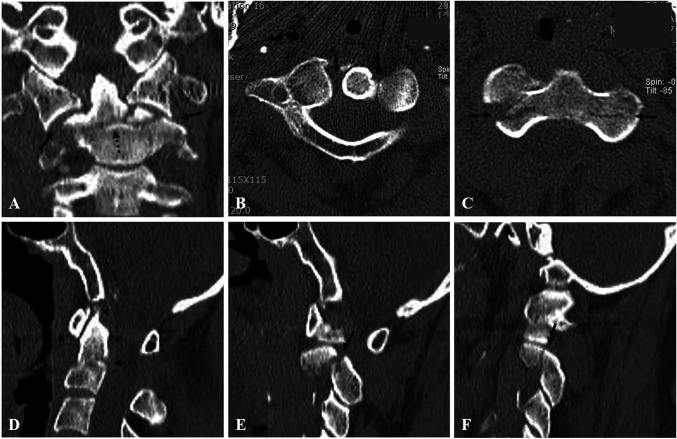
Coronal (A) CT (CT) scan showing a type 3 dens fracture (dotted dark arrow) and C1-2 lateral subluxation (dark arrows). Axial (B and C) CT scans showing asymmetry of C1-2 joints (dark arrows) and fractures of both lateral masses (dark arrows). Sagittal (D) CT scan showing a type 3 dens fracture with angulation (dotted dark arrow). Right and left parasagittal (E and F) CT scans showing fractures of both pars interarticularis (dark arrows), indicating a type 1 hangman fracture.
